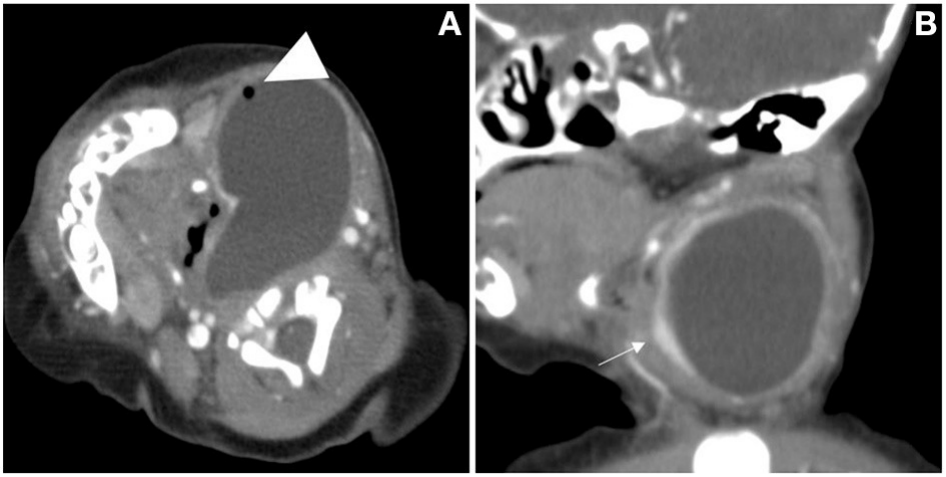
The cervical CT images of a 7-day-old girl. A cystic mass without septum was demonstrated in the left neck, small air bubbles can be seen inside (arrowhead), the wall of the cyst was thin and iso attenuation, and the border was well defined. The cystic mass, shaping as a “comma,” extended laterally into the retropharyngeal space and the airway was deviated (A). The left thyroid gland lobe was involved and pushed anteriorly on sagittal view (B, arrow).
